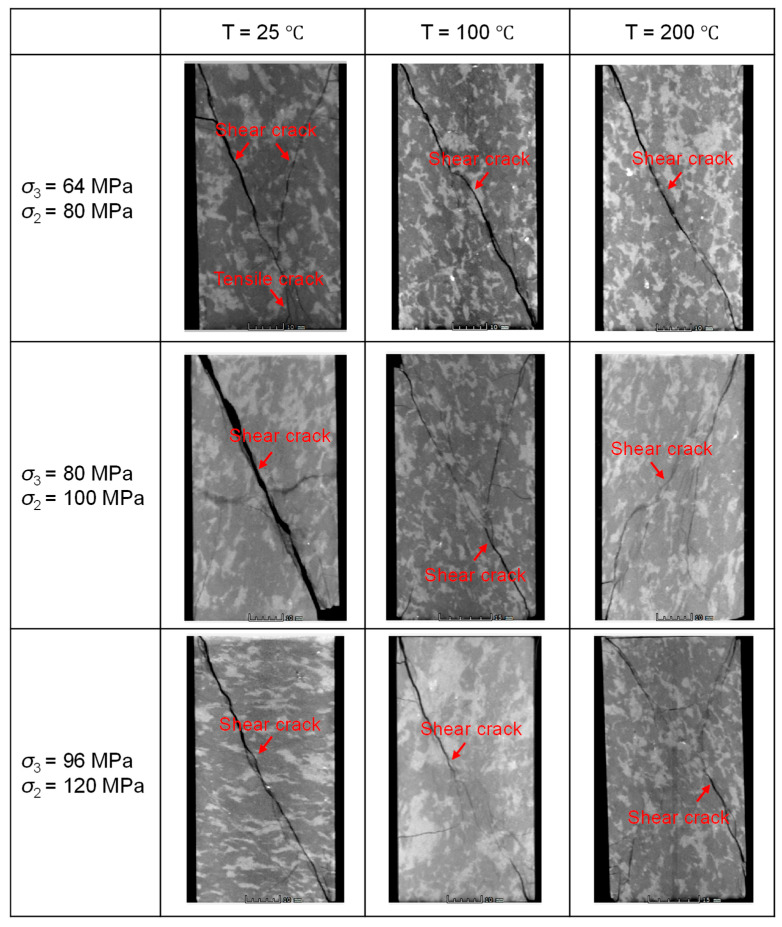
CT slices of granite after failure under different temperature conditions (The sample in the CT slice is 40 cm wide and 80 cm high).
